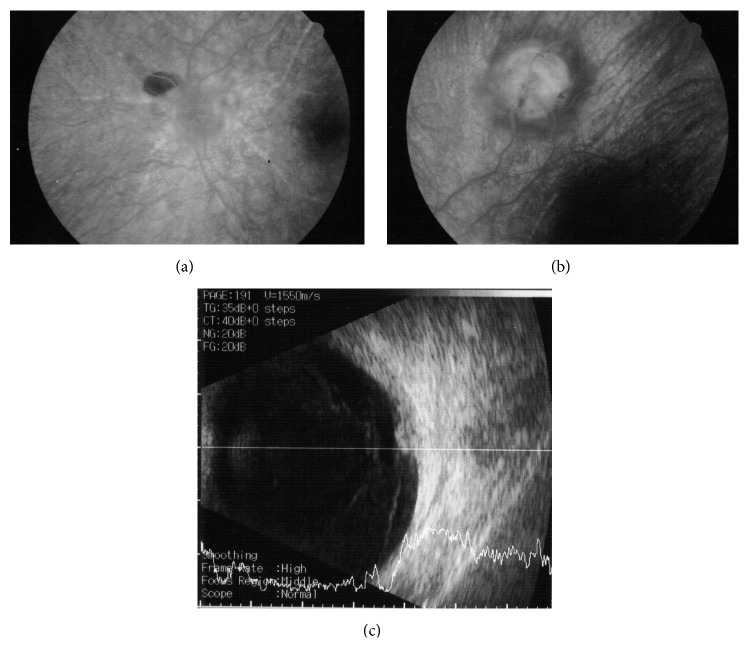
(a-b) Fluorescein angiography (FA) demonstrated background hyperfluorescence corresponding to the “sunset glow fundus” and hyper- and hypofluorescence of the RPE proliferating lesions. (c) A B-scan ultrasound of the larger peripheral RPE proliferations showed a dome-shaped mass 2 mm in height, high internal reflectivity, and a partial posterior vitreous detachment.
